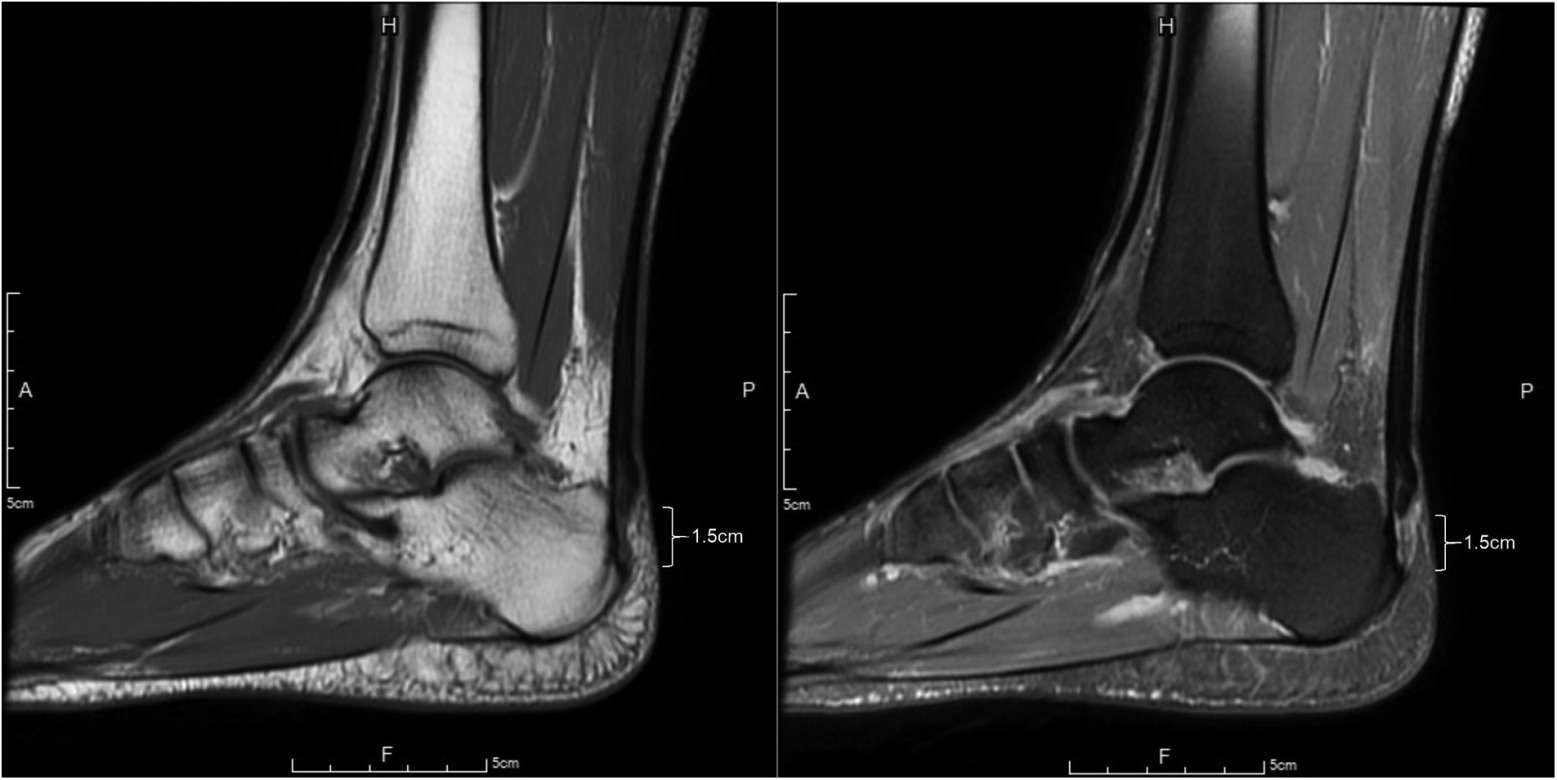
MRI images of the patient's Achilles tendon. Mid-sagittal view using T1-weighted imaging (left) and sagittal view using PD-FS imaging (right).
